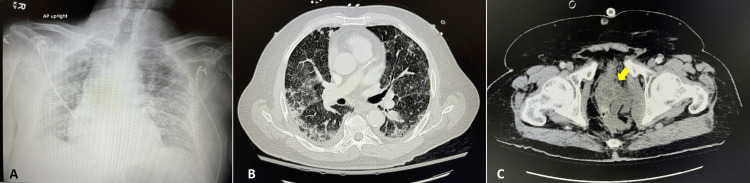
Radiography on admission. (A) Chest X-ray with bilateral diffuse interstitial and airspace opacities. (B) CT scan of the chest showing significant patchy mixed ground glass and consolidative opacities indicative of multifocal pneumonia. (C) CT scan of the abdomen with a complex multiloculated collection near the prostate extending along the lateral aspect of the rectum, which is concerning for neoplasm or abscess. CT, computed tomography
